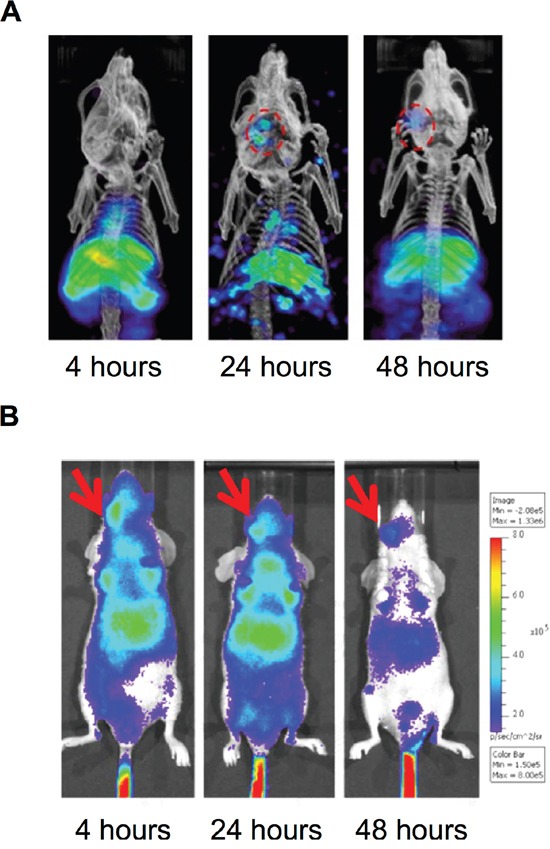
Accumulation of PEGylated ^188^Re-liposome in orthotopic HNSCC tumor lesion A. γ-rays emission of ^188^Re-liposome was detected in the HNSCC tumor lesion *in vivo* at different time points using nanoSPECT/CT imaging, Circles represented the region of interest (ROI); B. β particles emission caused Cerenokov bioluminescent imaging by ^188^Re-liposome was detected using the IVIS optical imaging system. The arrows indicated positions of implanted tumors.
